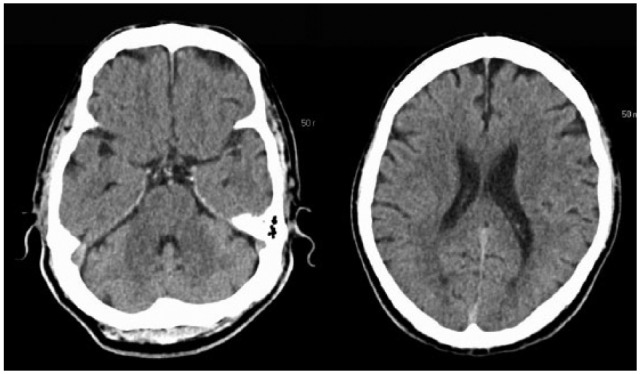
CT head done at the time of admission shows no ischemic stroke.
